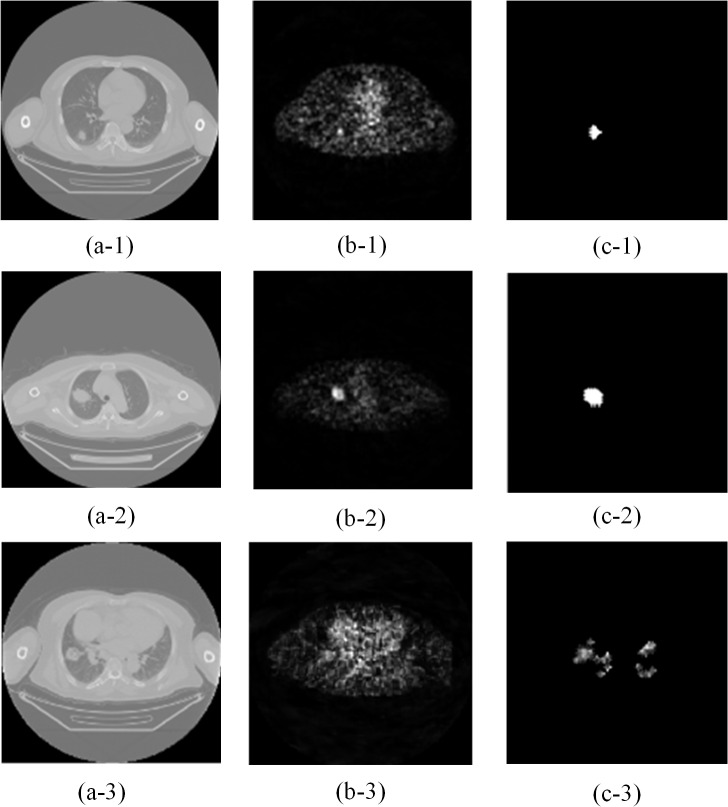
Results of nodule segmentation in different PET/CT images. (a-1, -2, and 3) CT images; (b-1, -2, and 3) corresponding PET images; (c-1, -2, and 3) results of segmentation.
